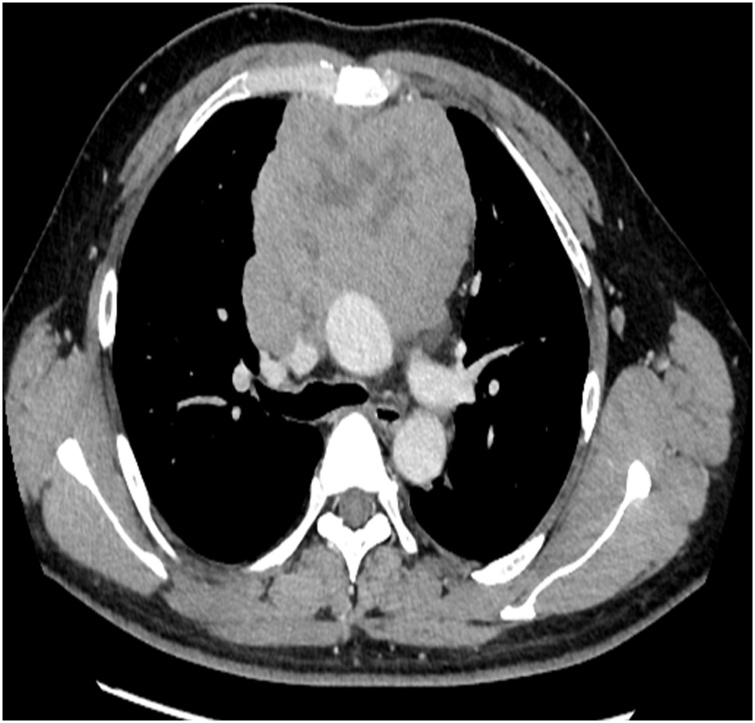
Chest computed tomography showing a large anterior mediastinal tumor and tracheal compression.
